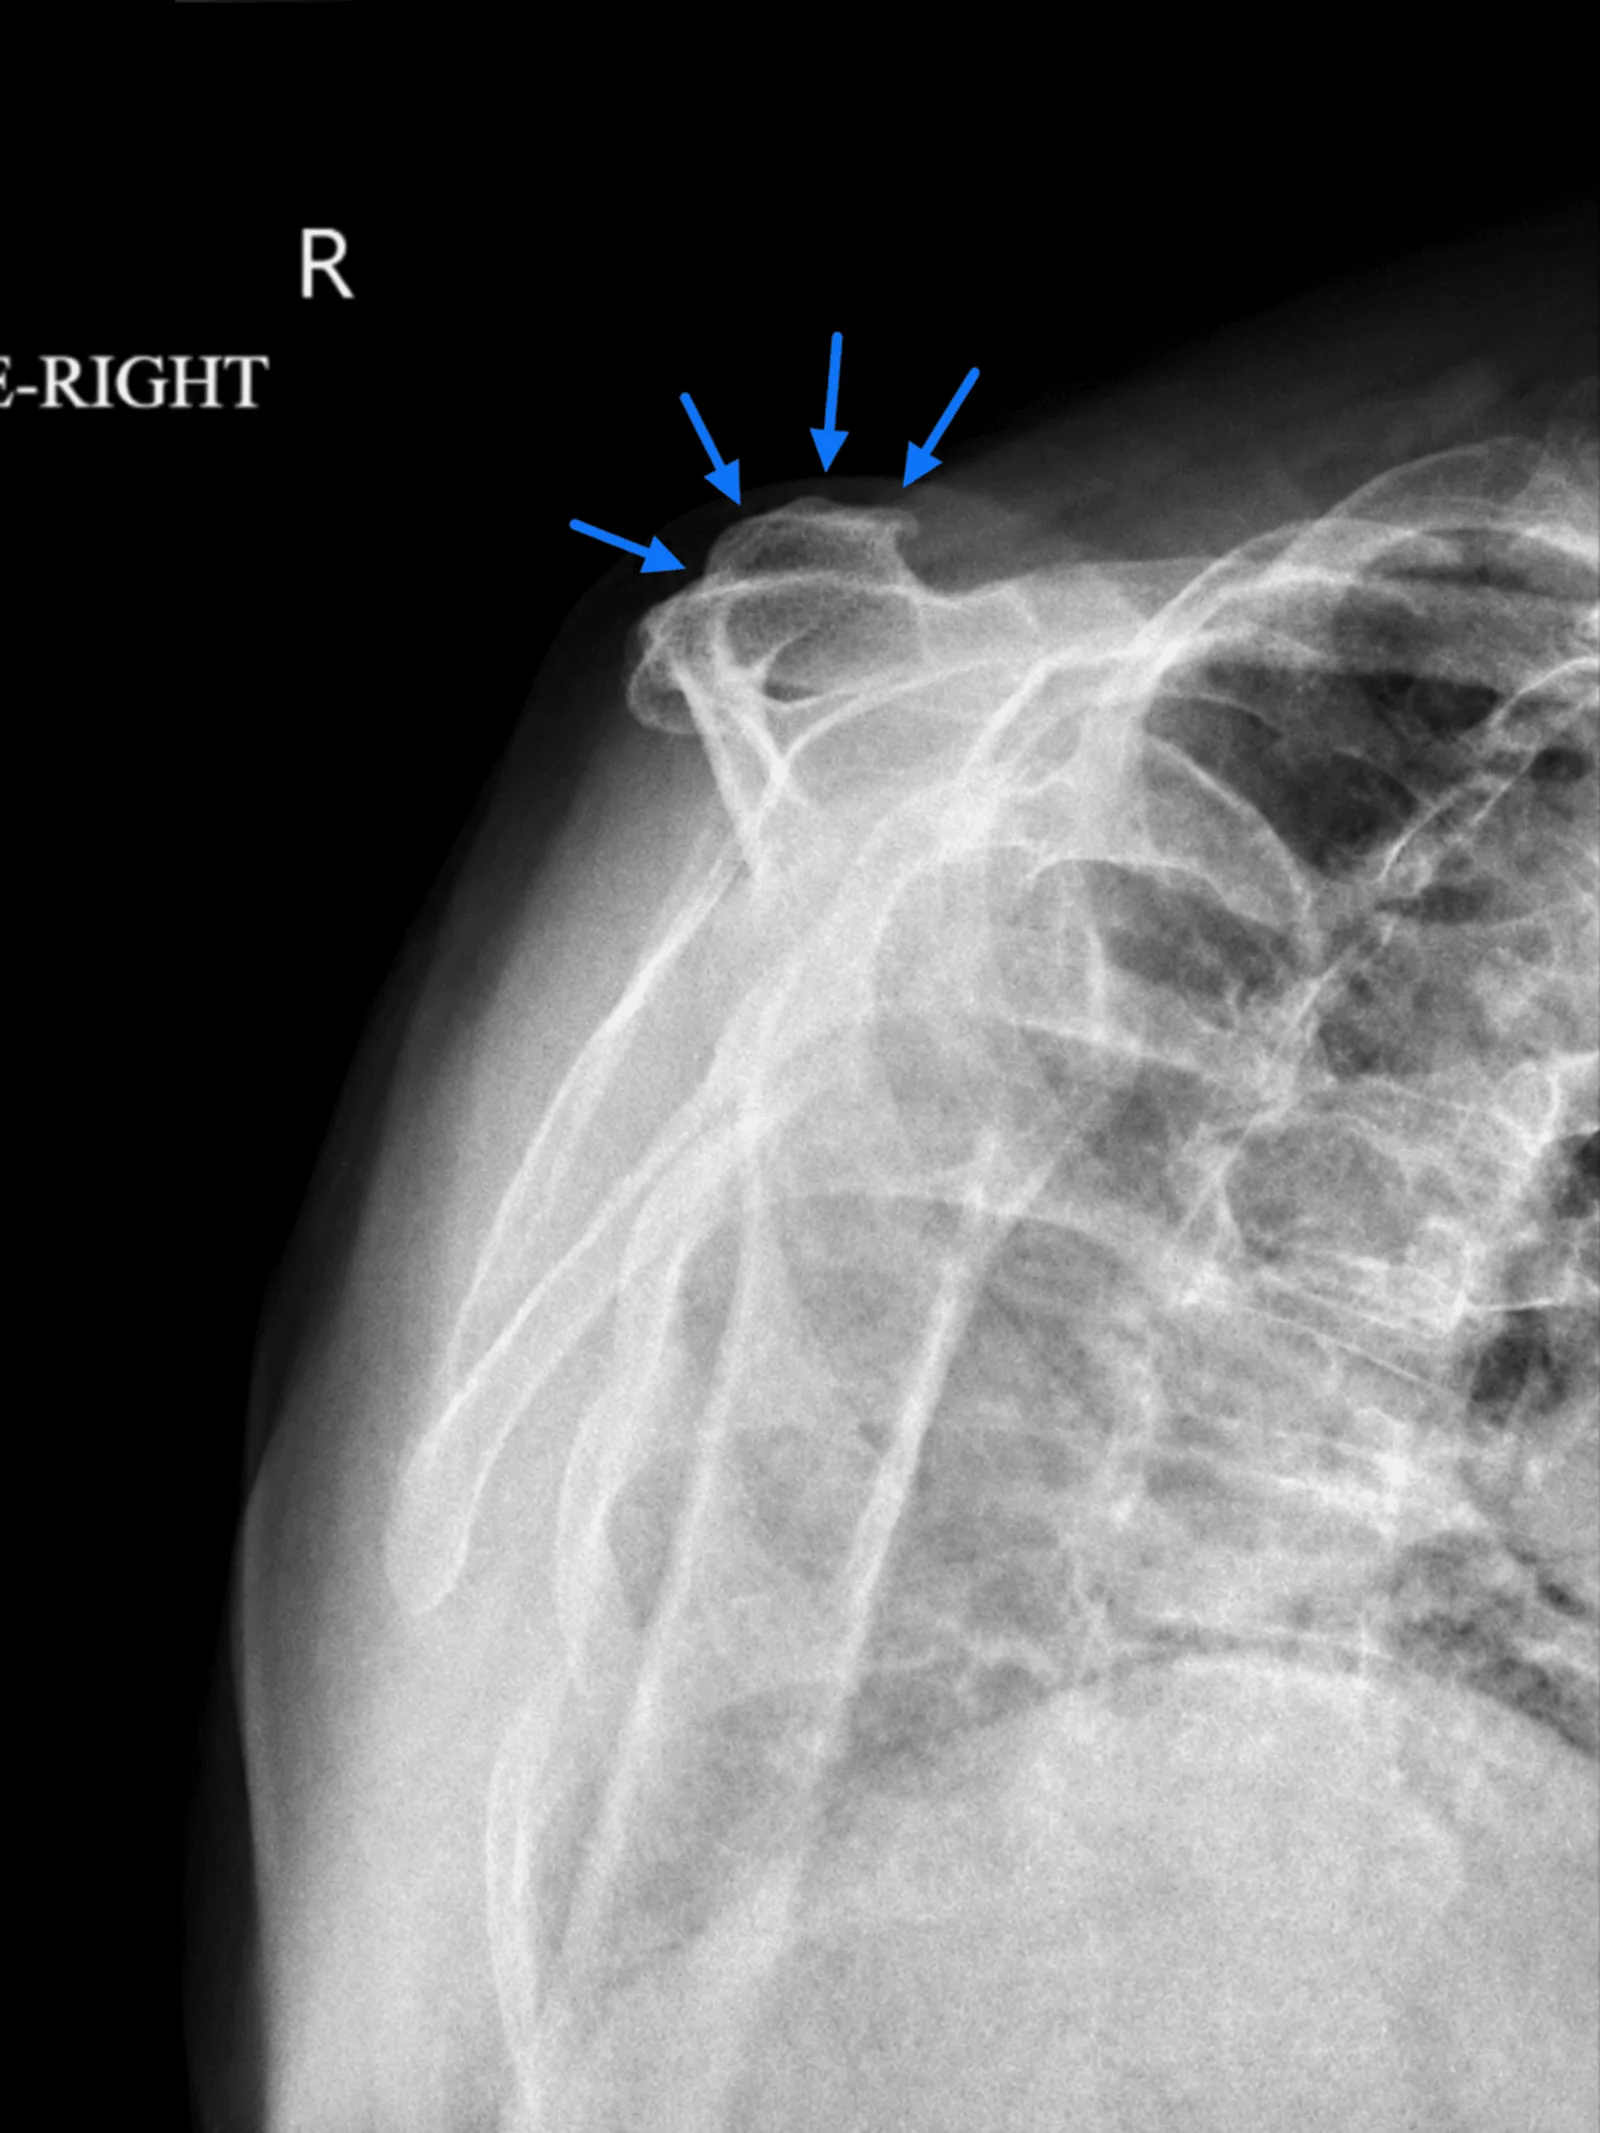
X-ray of the Right Shoulder X-ray of the right shoulder showing no acute fracture or dislocation and osteoarthritic changes at the acromioclavicular joint (blue arrows).
